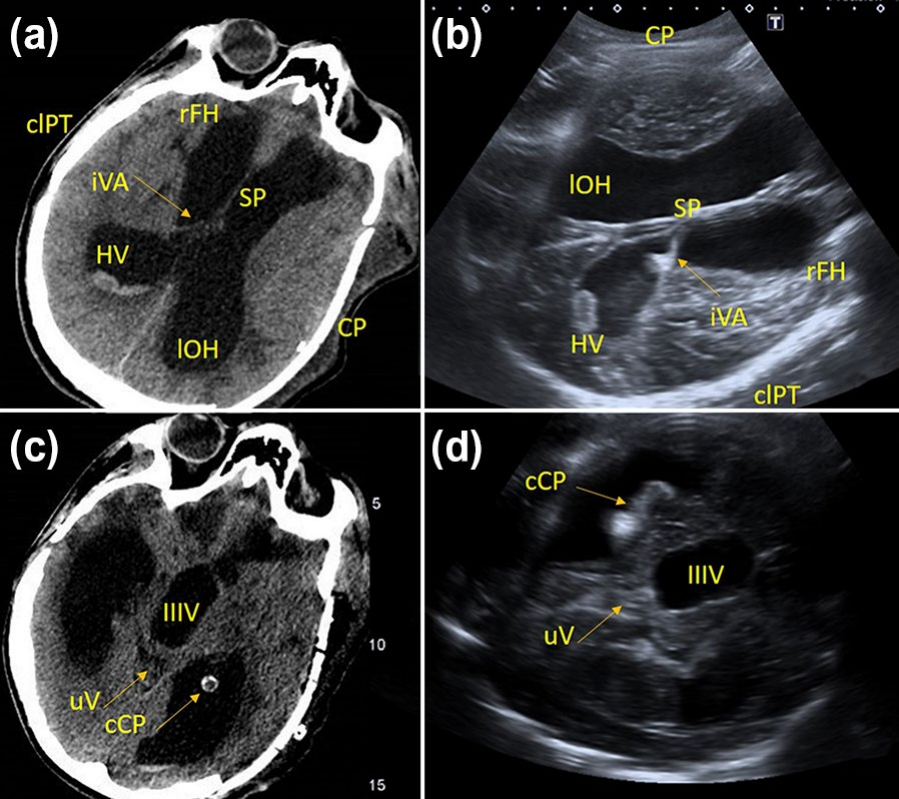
Postshunt evaluation at transcranial ultrasound (b, c) in comparison with CT (a, d). The course of catheter is clearly visible with transcranial ultrasound; ventricular size can be easily measured and monitored over time with bedside assessments. cCP, calcified choroid plexus, clPT, contralateral parietal teca, CP, cranioplasty, CT, computed tomography, HV, hemoventricle, IIIV, third ventricle, iVA, intraventricular adherences, lOH, left occipital horn, rFH, right frontal horn, SP, septum pellucidum, uV, upper vermis
